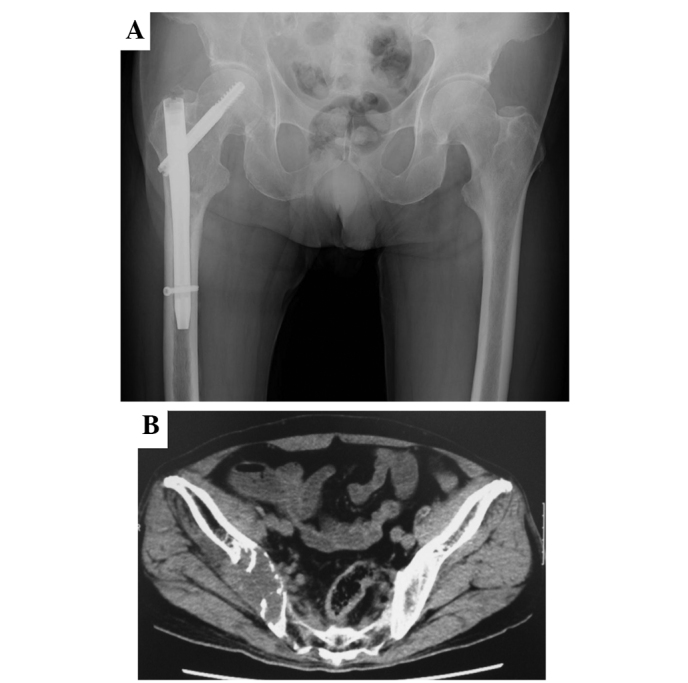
(A) Plain film at last eight months post-surgery showing the right femur with γ-nail fixation and no tumor progression. (B) Pelvic computed tomography confirming reduction of the mass in the ilium.
